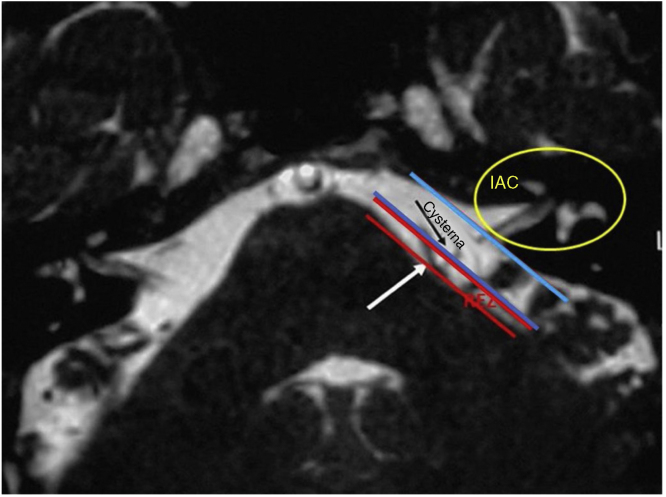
T2 weighted MRI showing the boundaries of the root entry zone (REZ) in the cerebellopontine angle and the internal auditory canal (IAC). Notice the conflict between the vessel (white arrow) and the facial nerve (black arrow) at the REZ.
